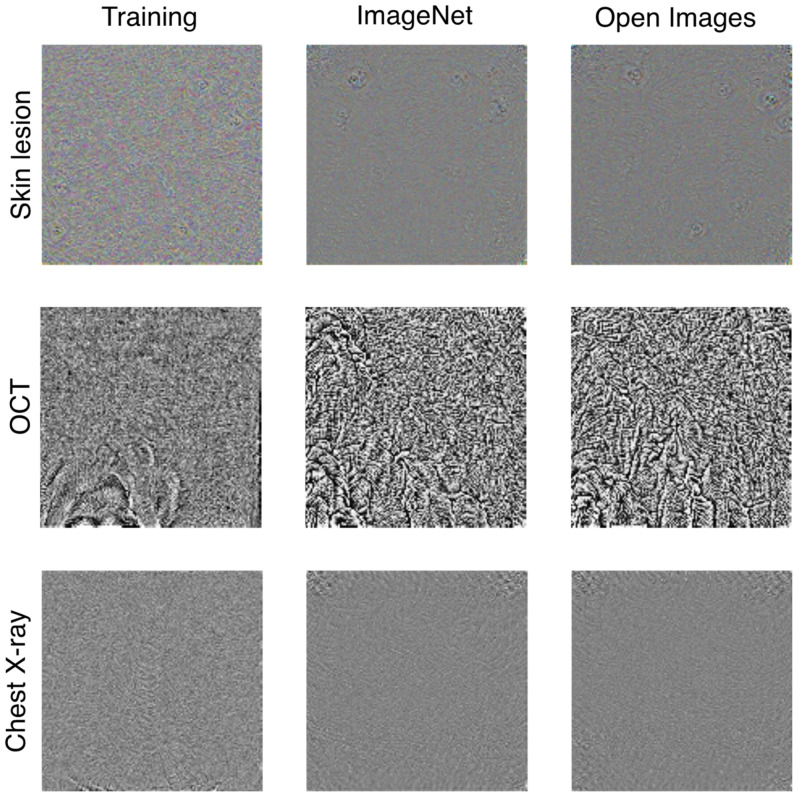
Visualization of targeted UAPs generated using training (medical image), ImageNet, and Open Images datasets against Inception V3 models for skin lesion, OCT, and chest X-ray image classifications. UAPs are visually emphasized for clarity; in particular, each UAP is scaled by a maximum of 1 and minimum of zero.
